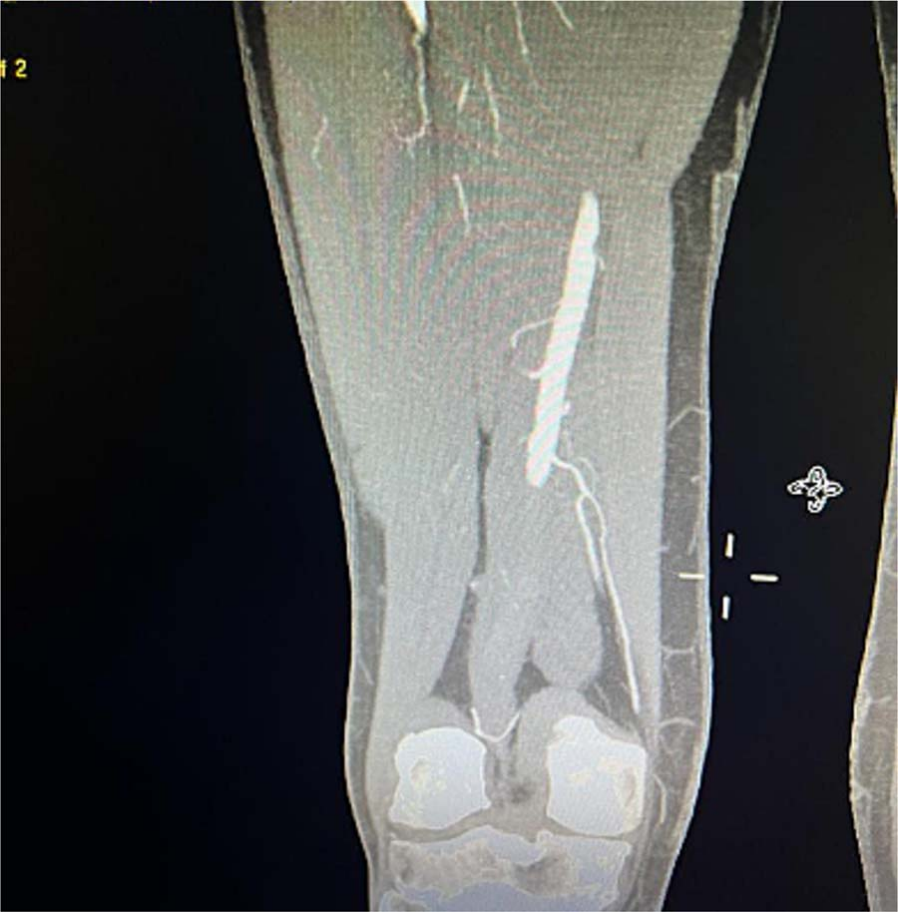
Coronal view of CTA scan showing right femoral artery embolic obstruction
